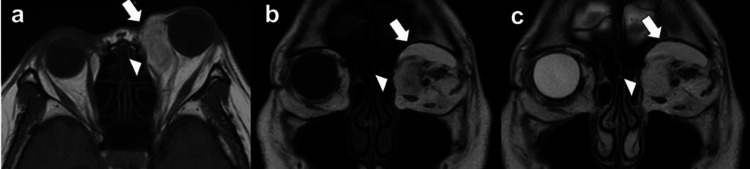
MRI images taken at the previous hospital 13 months after the first visit. a, b. Axial and coronal T1-weighted MRI images showing a homogeneous, high-intensity mass (arrow) and a newly developed heterogeneous appearance with mixed low and high-signal intensities (arrowhead); c. Coronal T2-weighted MRI image also showed both homogeneous area (arrow) and heterogeneous area (arrowhead) in the superomedial orbit.
